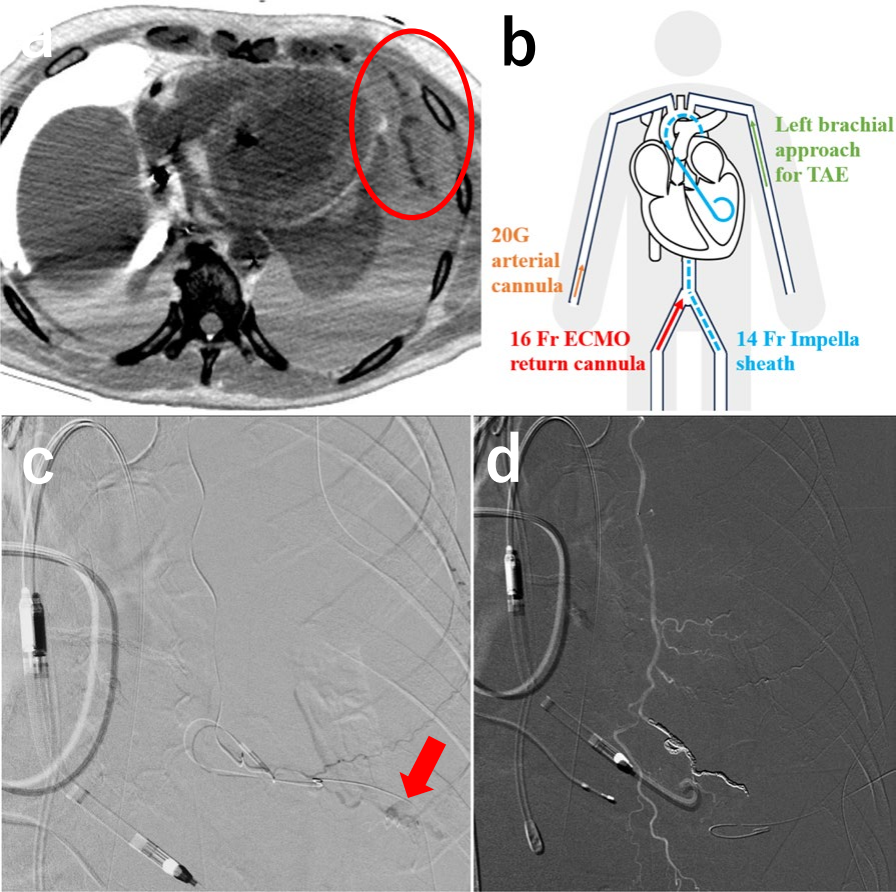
CECT and TAE on the 7th day of the patient’s hospitalization. a Arterial phase of thoracic CECT showing a left hemothorax and EV into the left thoracic cavity (circle). b The venoarterial extracorporeal membrane oxygenation (VA-ECMO) plus Impella (ECPELLA) circuit and the access route for TAE. c Approaching from the left brachial artery, a left internal thoracic angiography showing EV in the peripheral branch (arrow). d The bleeding source was embolized using a N-butyl-2-cyanoacrylate-Lipiodol mixture, and its lower intercostal branch was embolized with a metal coil to prevent bleeding from the collateral tract. Final angiography confirmed the disappearance of EV. CECT: chest contrast-enhanced computed tomography, TAE: transarterial embolization, EV: extravasation
